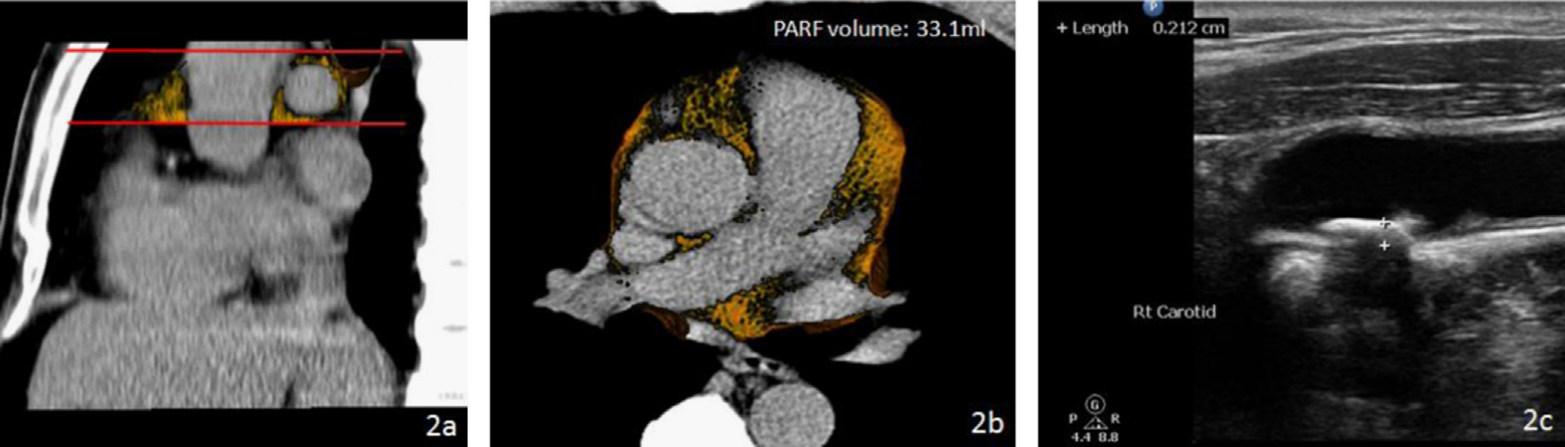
Comparison of two study subjects—one with a larger volume of peri-aortic root fat (PARF). Red lines indicate the 24 mm caudal to cranial limits of PARF. The orange color indicates adipose tissue surrounding the aortic root in sagittal (a) and axial (b) views. High resolution carotid ultrasound in the study subject with a larger volume of PARF (33.1 ml) showed greater intima-media thickness (2.1 mm) and plaque existence (c). (For interpretation of the reference to color in this figure legend, the reader is reffered to the web version of this article.)
